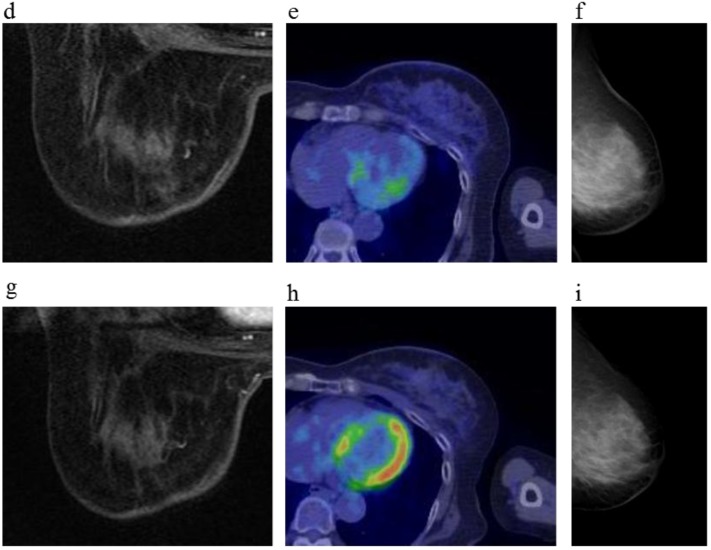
A 64-year-old female (case 3 in Table 1, Table 2 and Table 3) with left breast cancer (cT2N0M0). CE breast MRI, PET-CT and mammography revealed a breast tumor before KORTUC II therapy, the region of tumor was >20 mm (a–c). After the completion of KORTUC II, the tumor disappeared on the serial examination ((d–f): initial examination after KORTUC II treatment, (g–i): final examination), and no recurrence was identified. The findings of CE breast MRI seems to be most reliable among these three diagnostic modalities in terms of recognition of tumor disappearance.
